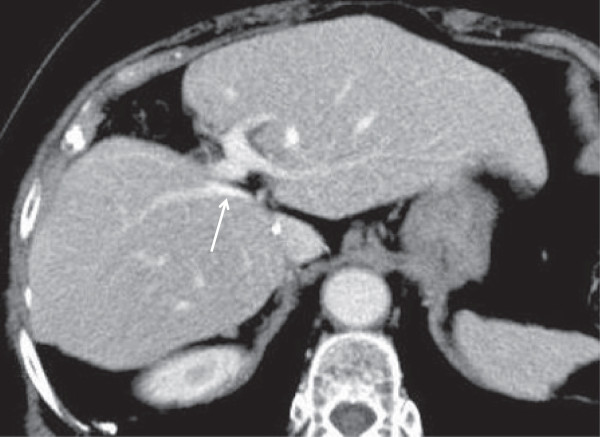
Postoperative CT revealed that the central bisections and caudate lobe are removed, the RHV (arrow) is exposed on the raw surface of the liver, and the IVC is exposed. IVC, inferior vena cava; RHV, right hepatic vein.
